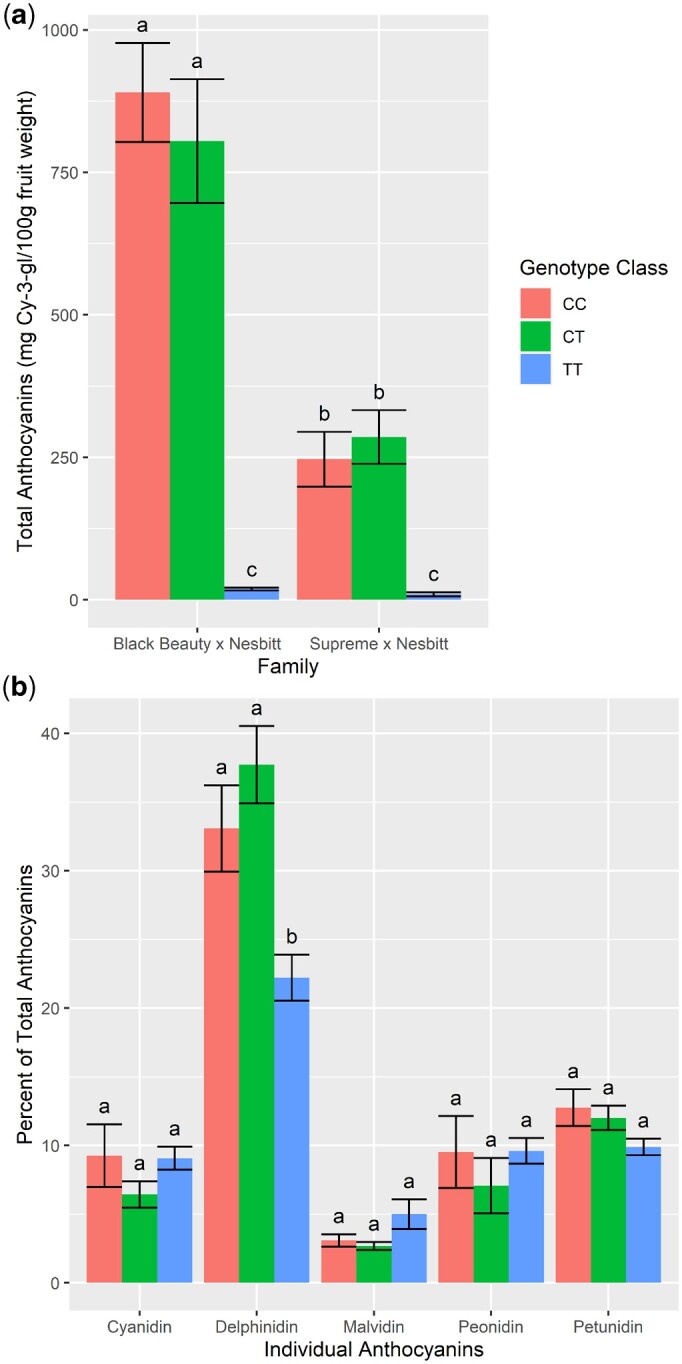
Anthocyanin profiling of postvéraison berry skins from black (CC & CT) and bronze (TT) muscadine genotypes from the “Supreme” × “Nesbitt” and “Black Beauty” × “Nesbitt” mapping populations. a) Total anthocyanin content from each population and b) Individual anthocyanins represented as an average of both populations. Error bars represent standard error of means.
